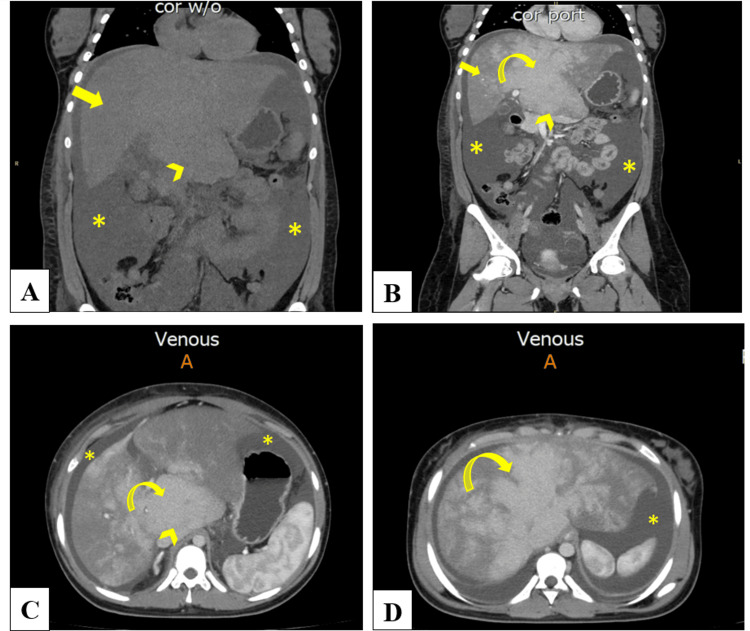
Contrast-enhanced CT showing findings characteristic of Budd-Chiari syndrome. (A) Non-contrast coronal CT showing hepatomegaly (arrow), caudate lobe hypertrophy (arrowhead), and ascites (asterisk). (B) Coronal portal venous phase with contrast showing hepatomegaly (arrow), caudate lobe hypertrophy (arrowhead), ascites (asterisk), and central-predominant enhancement with peripheral hypoenhancement (curved arrow), consistent with hepatic venous outflow obstruction. (C, D) Axial portal venous phase images showing caudate lobe hypertrophy (arrowhead), ascites (asterisk), and a mottled “nutmeg” liver appearance (curved arrow) reflecting centrilobular congestion. These combined findings - hepatomegaly, ascites, caudate lobe hypertrophy, central enhancement, and “nutmeg” pattern - are characteristic of Budd-Chiari syndrome.
